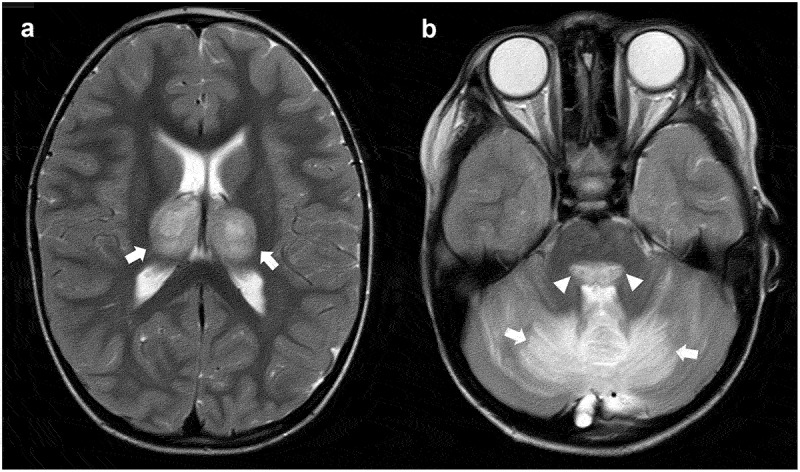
Magnetic resonance imaging in a 3 year old presenting with a viral prodrome followed by rapid neurological deterioration. T2-weighted axial images demonstrating the characteristic thalamic lesions (Panel A, arrows), alongside more widespread changes in brainstem (Panel B, arrow heads) and cerebellum (Panel B, arrows) seen in acute necrotizing encephalopathy.
